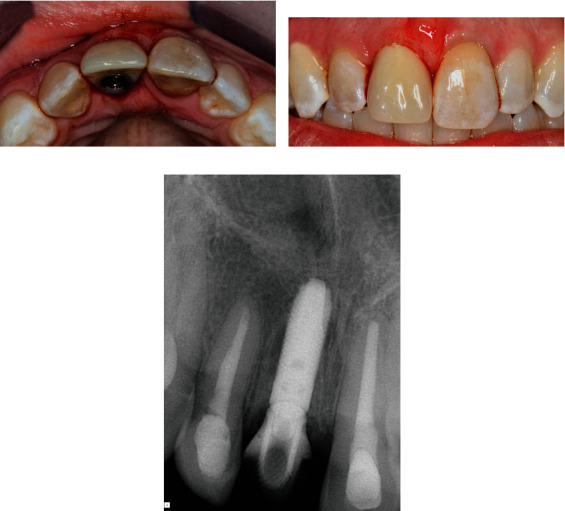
A customized screw-retained provisional crown was made chairside immediately after implant placement. (a) Occlusal view of the provisional crown showing the location of the implant at the cingulum region. (b) Facial view of the provisional crown displaying its subgingival contour conformed to support the soft tissue emergence profile. (c) A periapical radiograph was taken immediately after provisional crown restoration.
